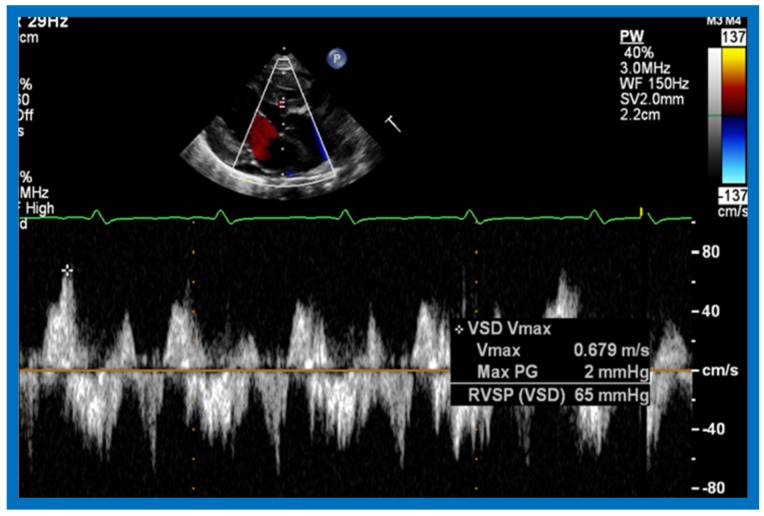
Selected video frame of continuous wave Doppler across the ventricular septal defect of the same baby shown in Figure 22. Low velocity flow across the ventricular septal defect suggests that the defect is nonobstructive. Reproduced from Rao P.S. [39].
